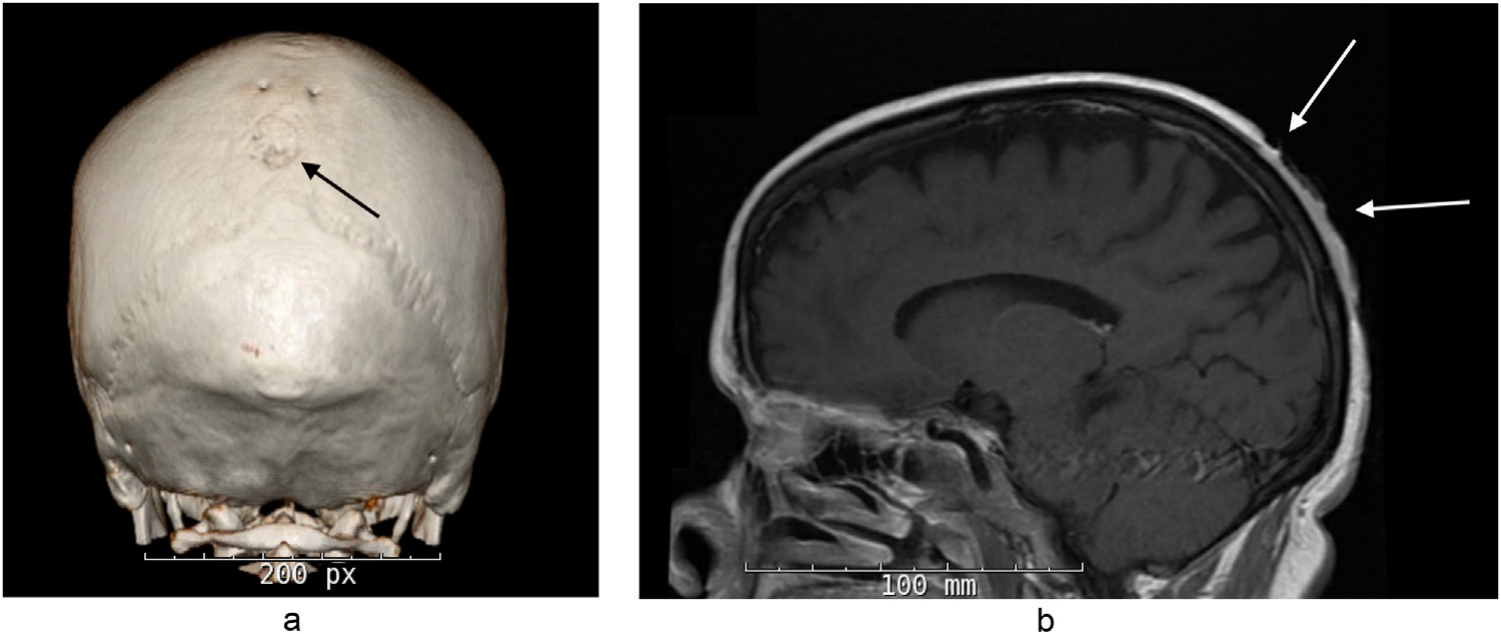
a: CT scan converted to 3D model depicting bone erosions of the cranium. b: MRI demonstrating cranial invasion.
